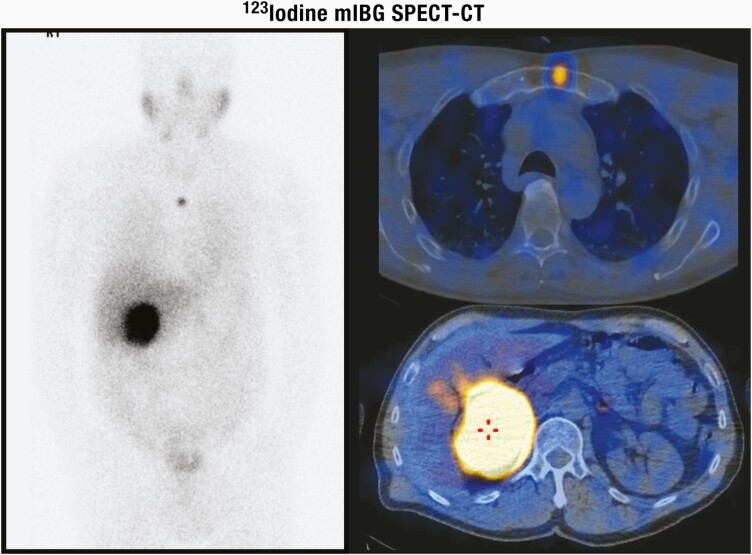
Iodine-123 metaiodobenzylguanidine SPECT-CT. Anterior half-body planar view and axial fused SPECT-CT images in a patient with a large MIBG-avid right adrenal pheochromocytoma with an unsuspected tracer-avid bone metastasis within the manubrium.
